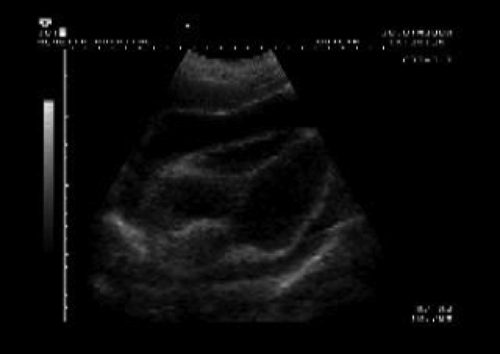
Ultrasound post-pericardiocentesis for the patient, showing persistent large effusion but improved right ventricular filling.
